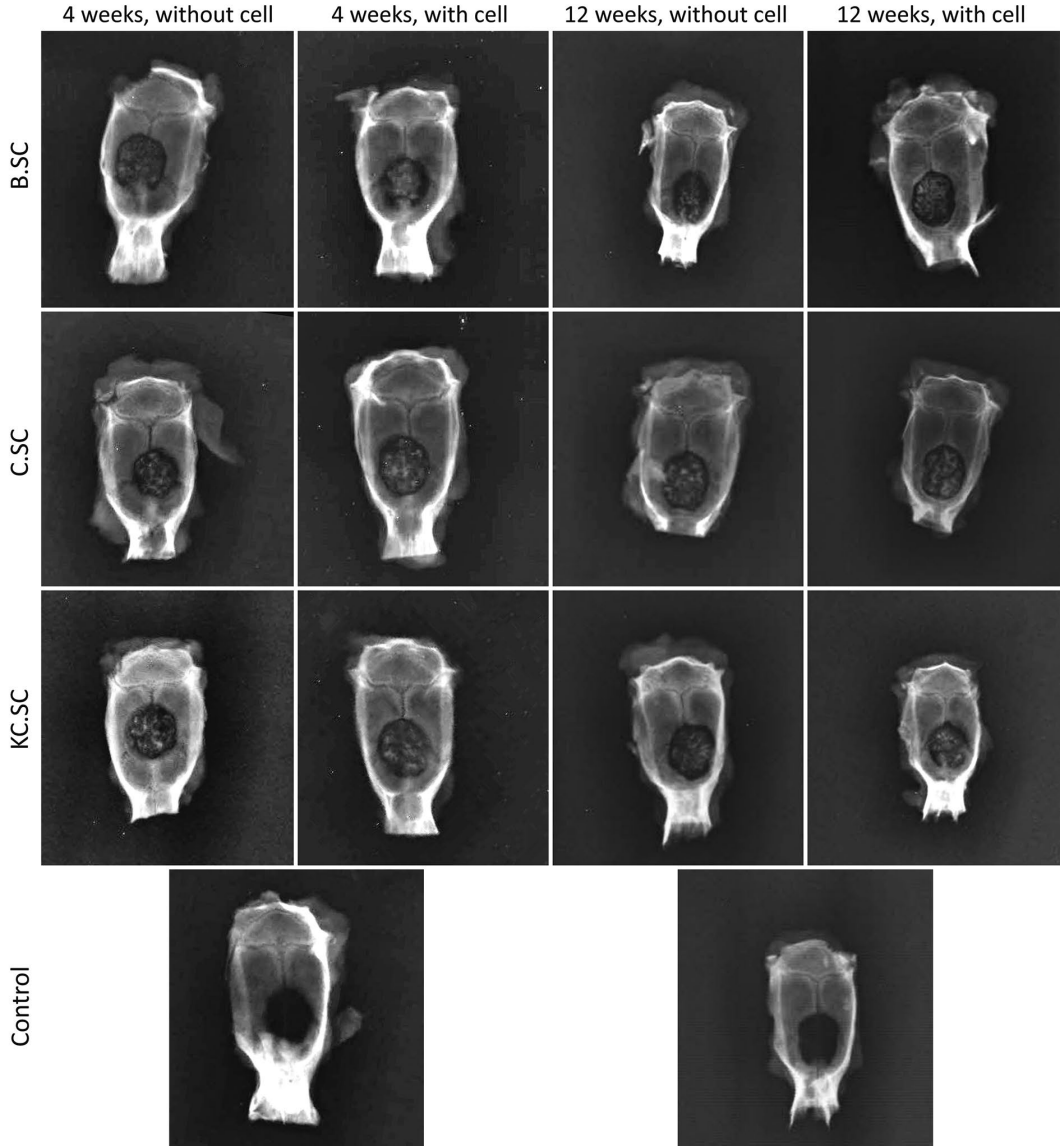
Reprehensive radiographs after 4 and 12 weeks post-implantation. B.SC: bare scaffold, C.SC: Zein-coated scaffold, KC.SC: Kaempferol-loaded C.SC, B.SC + cell: bare scaffold + BMSC, C.SC + cell: Zein-coated scaffold + BMSC, KC.SC + cell: Kaempferol-loaded KC.SC + BMSC.
